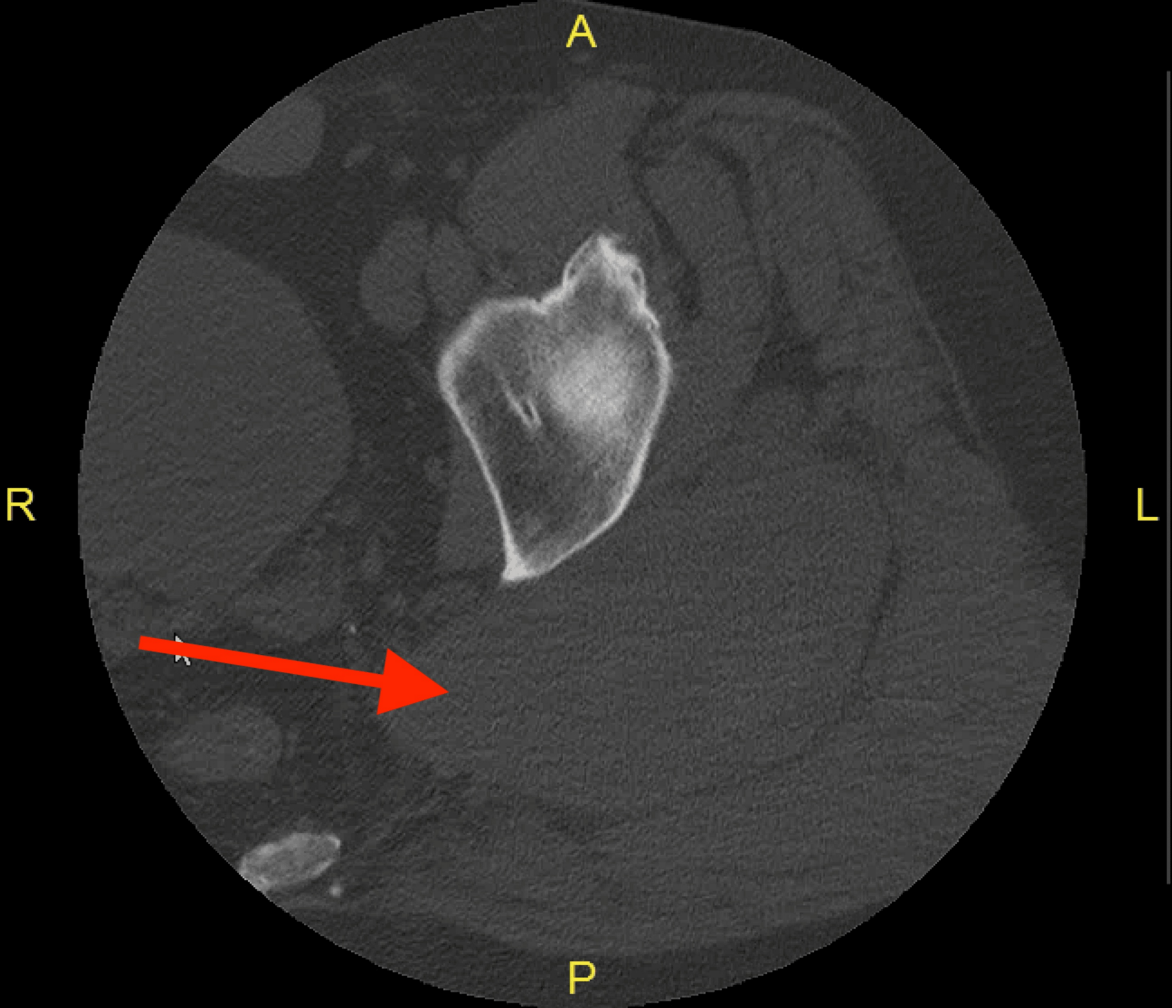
CT of the left lower extremity (axial view) There is no evidence of an acute fracture. Diffusely edematous left piriformis with a large intramuscular hematoma (red arrow) measuring 7.4 x 4 cm.
